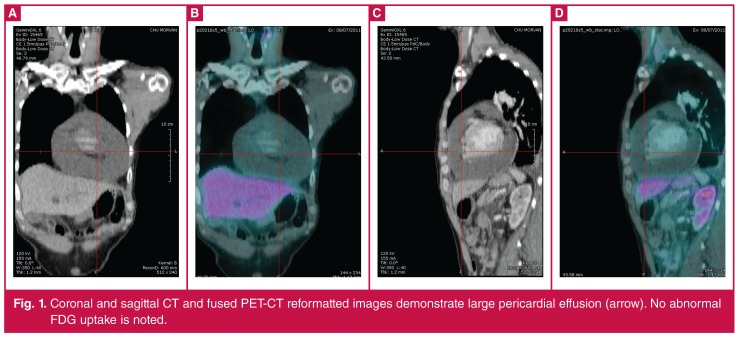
Coronal and sagittal CT and fused PET-CT reformatted images demonstrate large pericardial effusion (arrow). No abnormal FDG uptake is noted.
